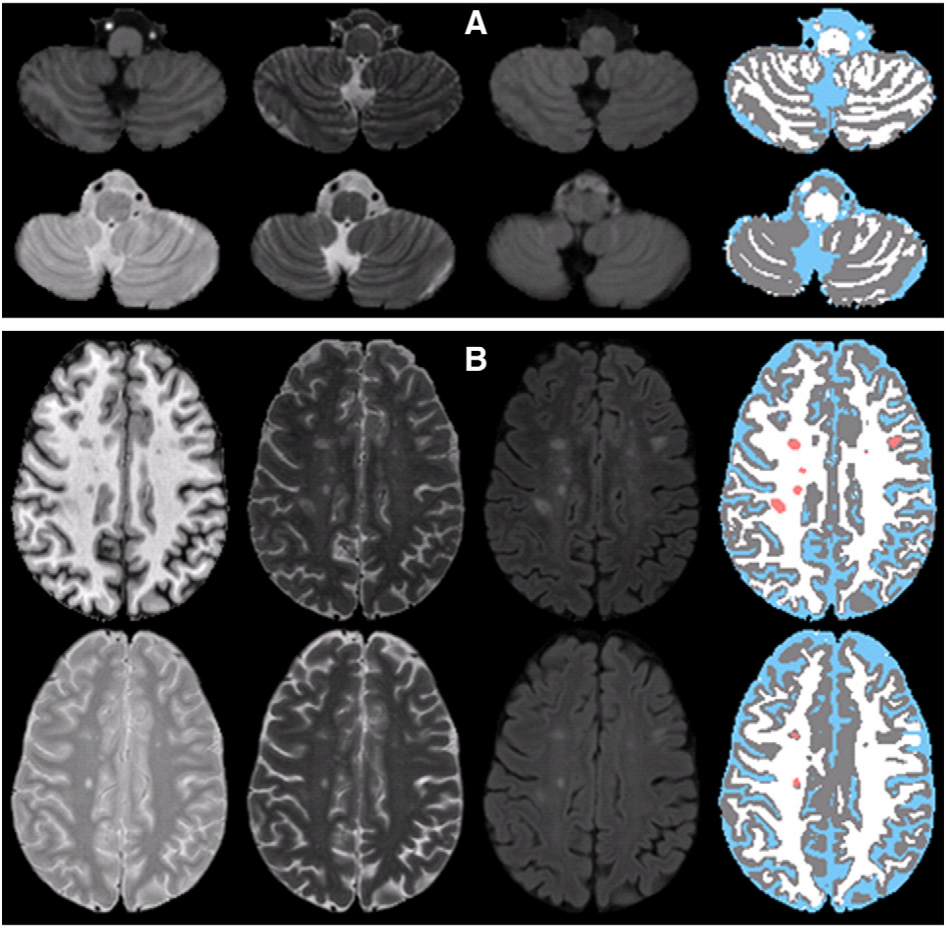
Demonstration of segmentation using the proposed technique on 3D images (T1, T2, and FLAIR) (top row) and using the technique proposed by Sajja et al. (2006) on 2D images (PD, T2, and FLAIR) (bottom row): (A) a section of the cerebellum and (B) the semiovale region. Color scheme is same as Fig. 2.
